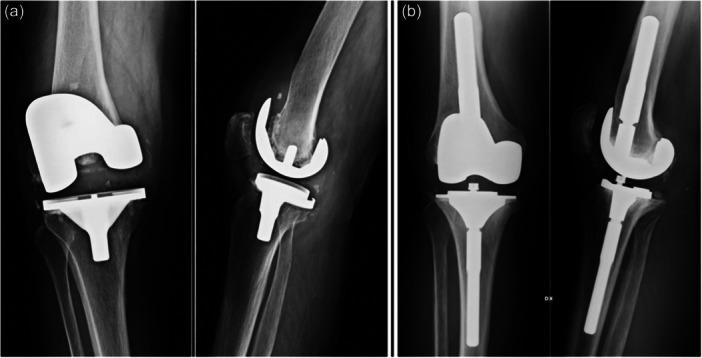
Preoperative and postoperative radiographic evaluation of rTKA in a 63 year old men. Anteroposterior and lateral radiographs of the right knee showing (a) preoperative failure due to aseptic loosening of the femoral component associated with an AORI type IIa bone defect and (b) postoperative appearance after rTKA with the use of K‐Mod revision medial pivot implant. Postoperative images demonstrate restoration of limb alignment and implantation of revision components with femoral and tibial stems, providing stable fixation and appropriate alignment. No radiolucent lines are visible at the 4‐year radiographic follow‐up. AORI, Anderson Orthopaedic Research Institute; rTKA, revision total knee arthroplasty.
